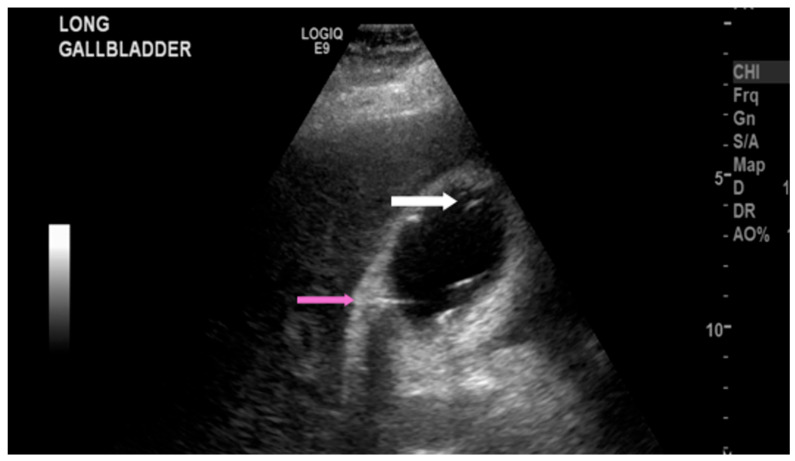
Right upper quadrant ultrasound of a 52-year-old female human immunodeficiency virus patient with emphysematous cholecystitis. Foci of air in the gallbladder lumen (white arrow) and intramural air (pink arrow) were demonstrated.
